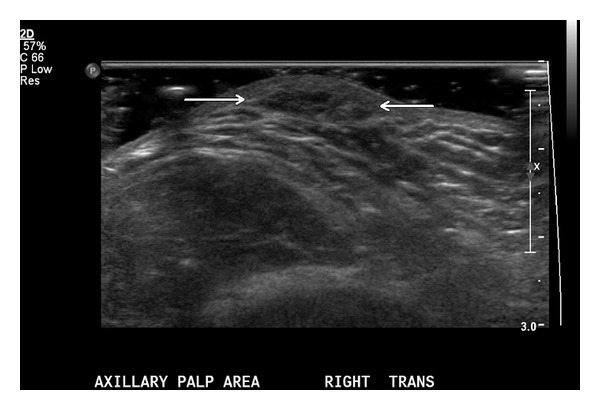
Representative ultrasound imaging of the right axilla demonstrates the area of palpable abnormality an approximately 1.2 cm oval, hypoechoic solid-appearing lesion. No capsule or posterior acoustic enhancement was evident. No vascularity was identified within the lesion on color Doppler interrogation and the adjacent vessels within the axilla were compressible and patent.
